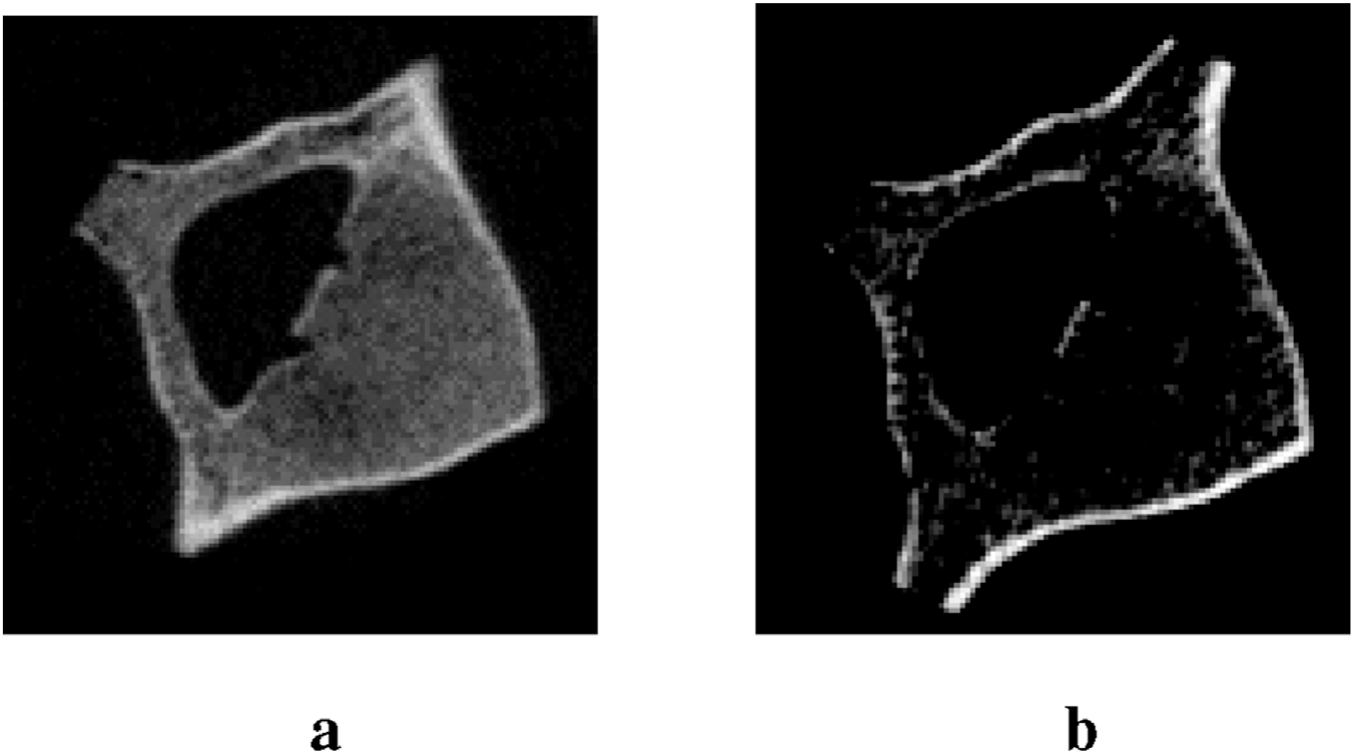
CT scans of vertebra #3 showing (a) scans before calibration and (b) scans after calibration.
